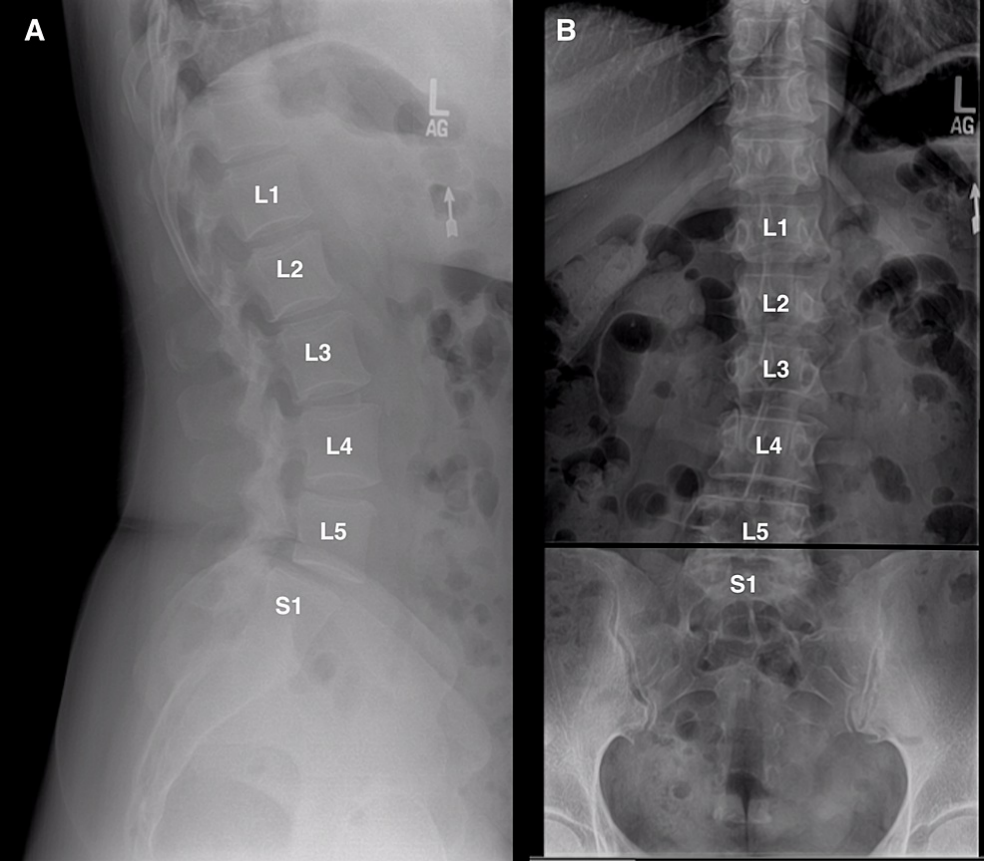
Lateral (A) and anterior–posterior (AP) (B) radiographs showing where the iliac crest is sitting right at the L5 vertebral column (black line), along with the lumbarized S1.
